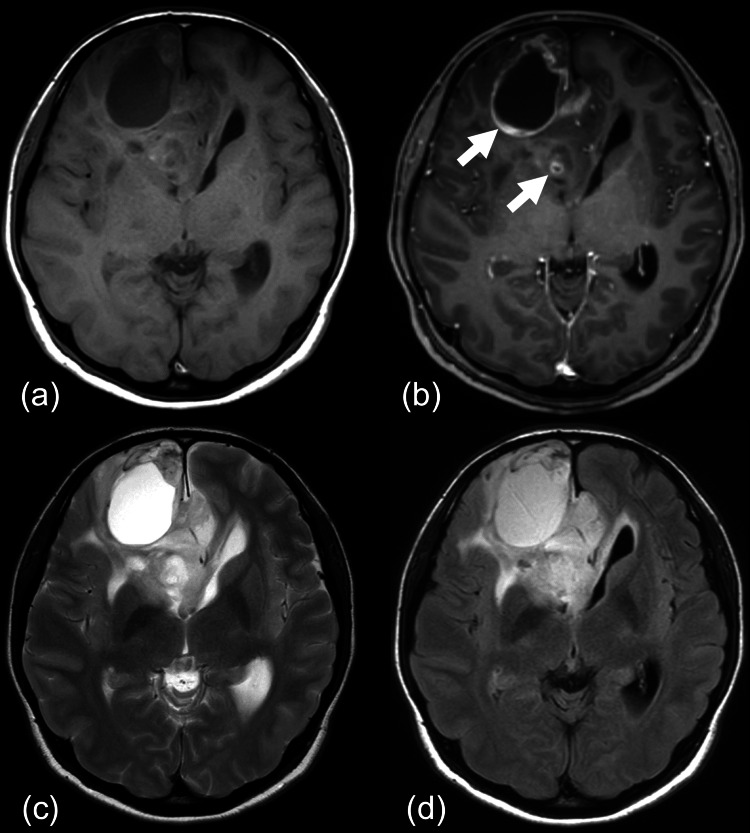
MRI scans Pre- and post-contrast T1-weighted images demonstrate irregular ring-enhancing lesions (arrows), with mass effect and involvement of the corpus callosum (a, b). MRI reveals a necrotic lesion in the right frontal lobe, with an ill-defined T2-hyperintense region (c, d). MRI, Magnetic resonance imaging
